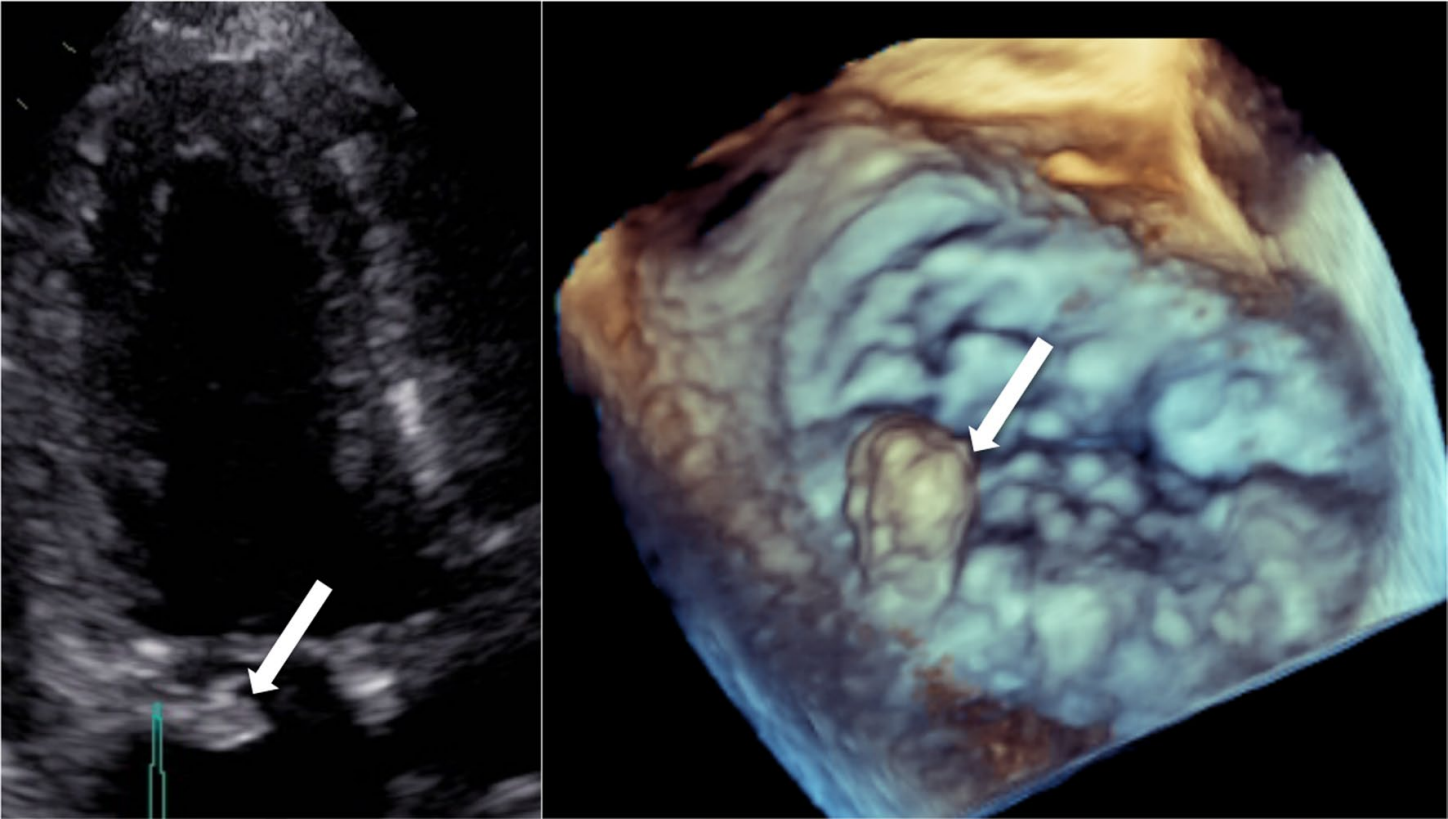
Transthoracic echocardiography showing 16 × 9 mm mobile vegetation of the posterior mitral valve leaflet
